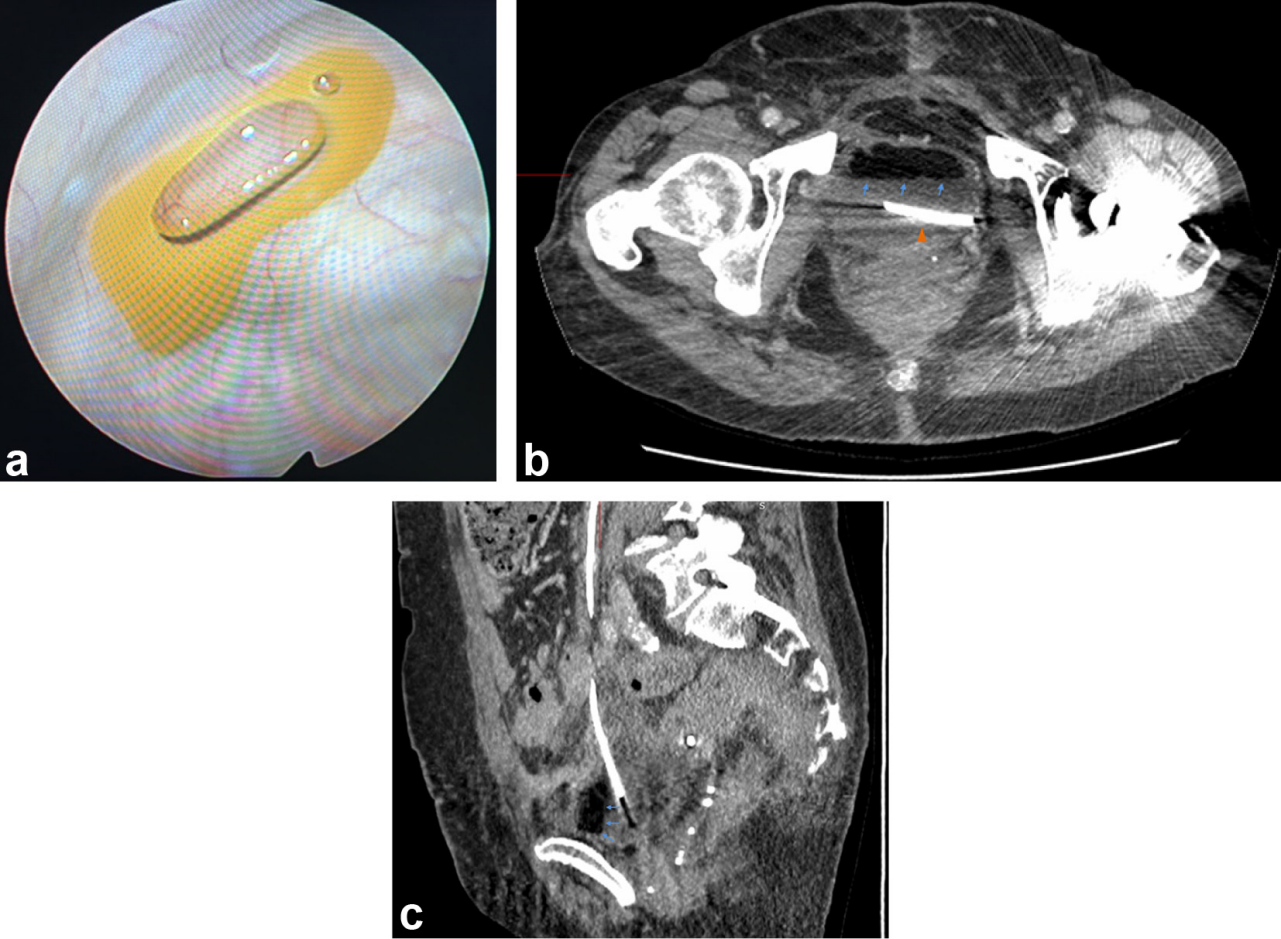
(a) Cystoscopic image demonstrating macroscopic fat in urinary bladder fundus. (b)Axial CT image at 6 months demonstrating intravesical fat-fluid level (arrows), correlating with the cystoscopic finding. Ureteric stent also noted (arrowhead). (c).Sagittally reconstructed CT image at 6 months demonstrating intravesical fat in the non-dependent position (arrows).
